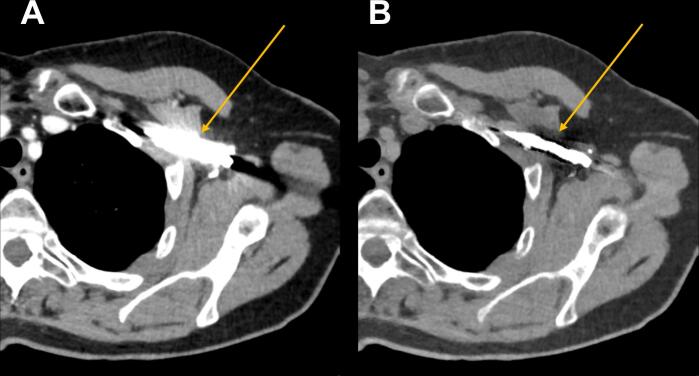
Example of the reduction of the artefact due to the high iodine concentration in the axillary and subclavian veins (arrow). A) Virtual mono-energetic image at 70 keV equivalent to a conventional CT image acquired at 120 kV. B) Virtual mono-energetic image at 190 keV leading to a smaller artefact, which could improve visualization of the adjacent lymphatic tissue. Both images are displayed at window width 350 and window level 40.
